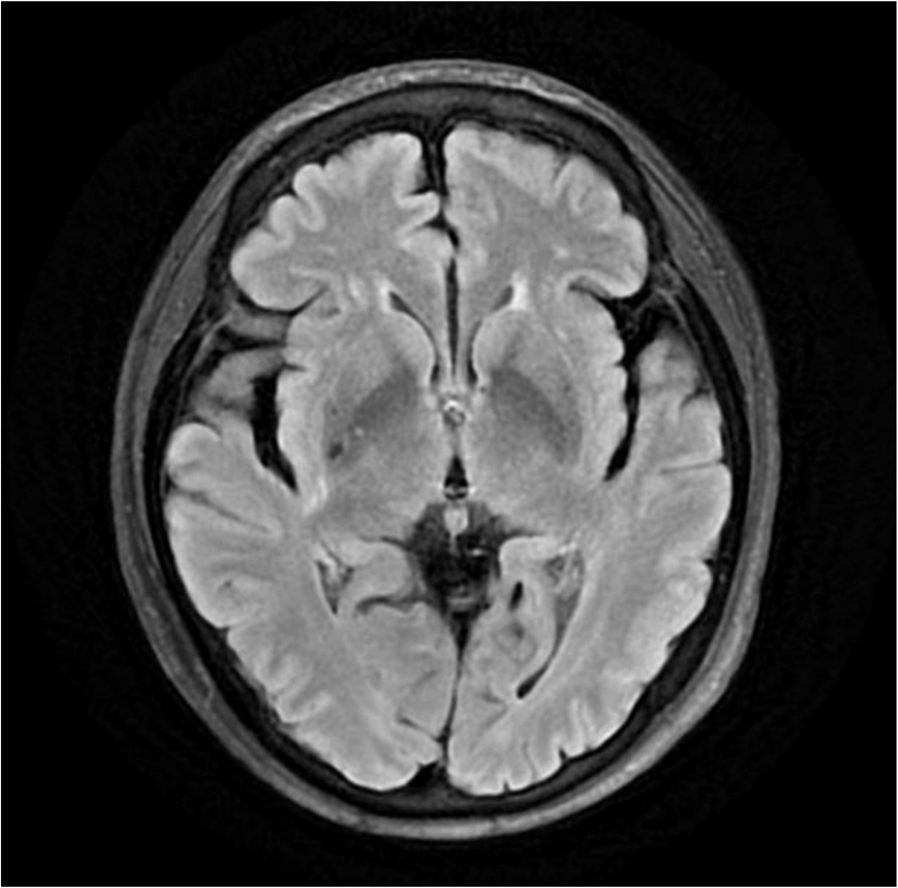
T2 magnetic resonance imaging showing old hemorrhagic infarct in the right basal ganglia
